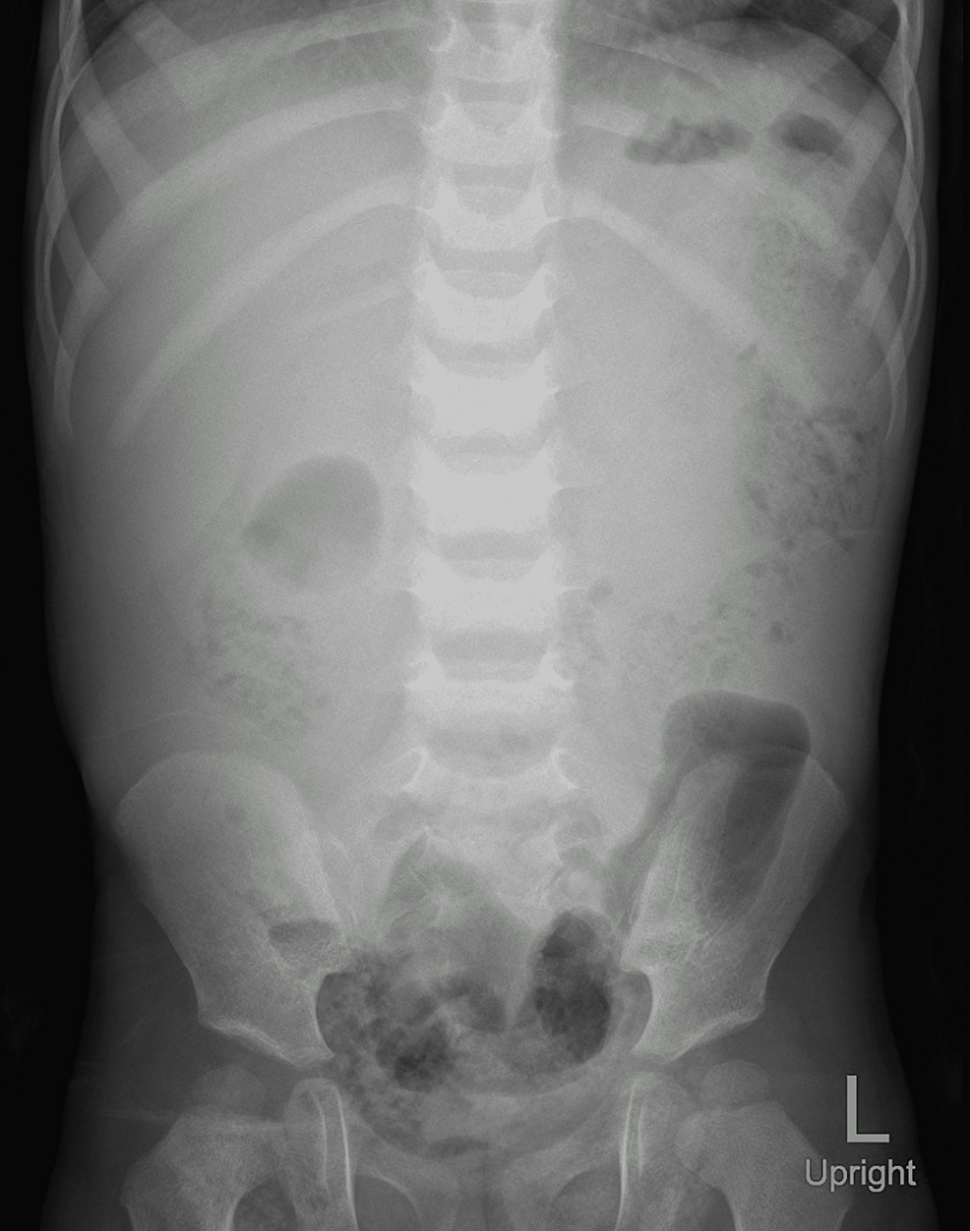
Digital X-ray of the abdomen in upright position revealing no evidence of air/fluid level
